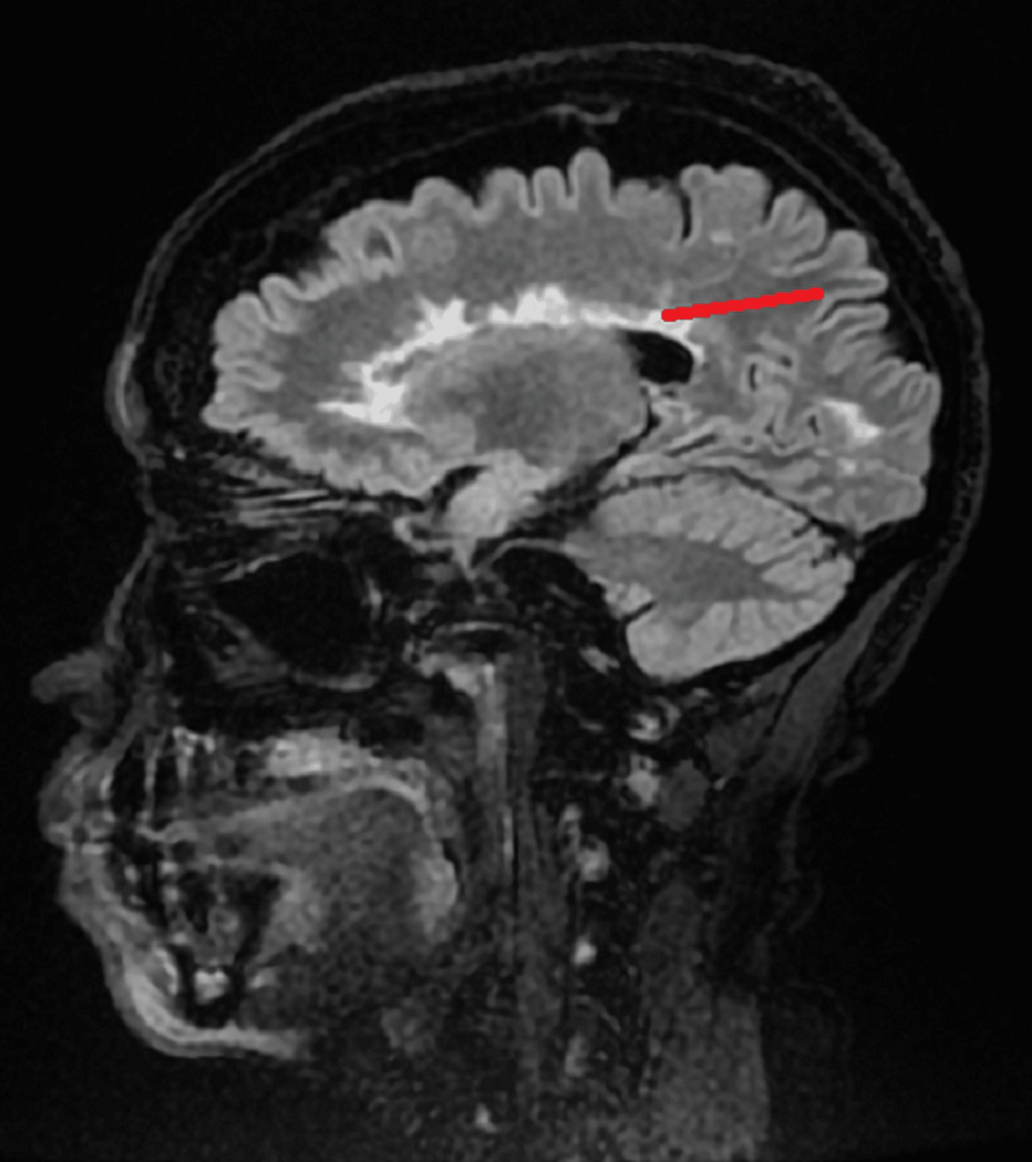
Sagittal view of brain MRI The red line points to Dawson’s finger on the brain MRI.
